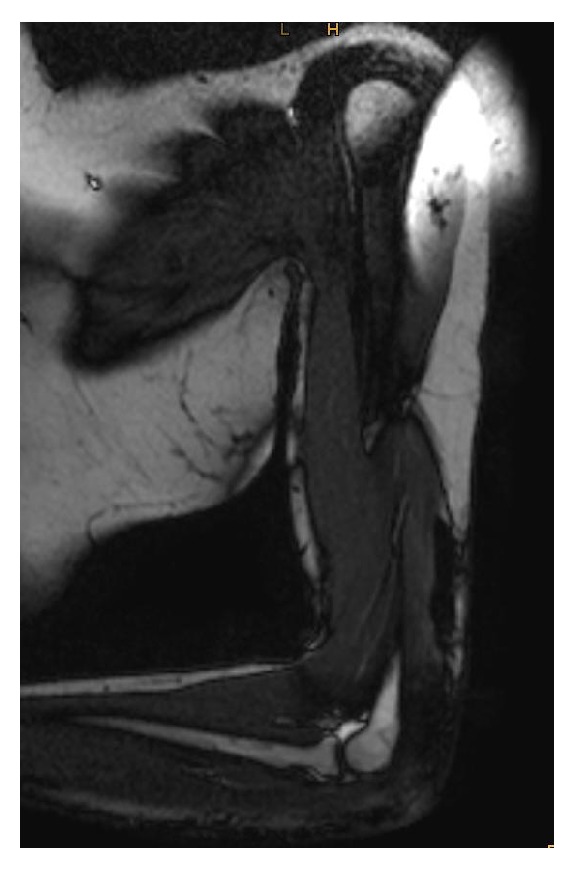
Sagittal T1 MRI scan of the right elbow of a 24-year-old woman with calcinosis universalis in juvenile Dermatomyositis with circumscribed areas of decreased signal intensity. No intra-articular effusion.
